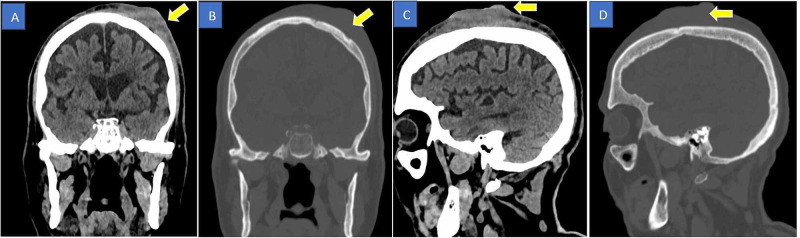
Plain CT head scan – coronal (A and B) and sagittal sections (C and D) The images show a left frontoparietal lesion with dermal and epidermal involvement causing bony erosion (yellow arrows) CT: computed tomography
